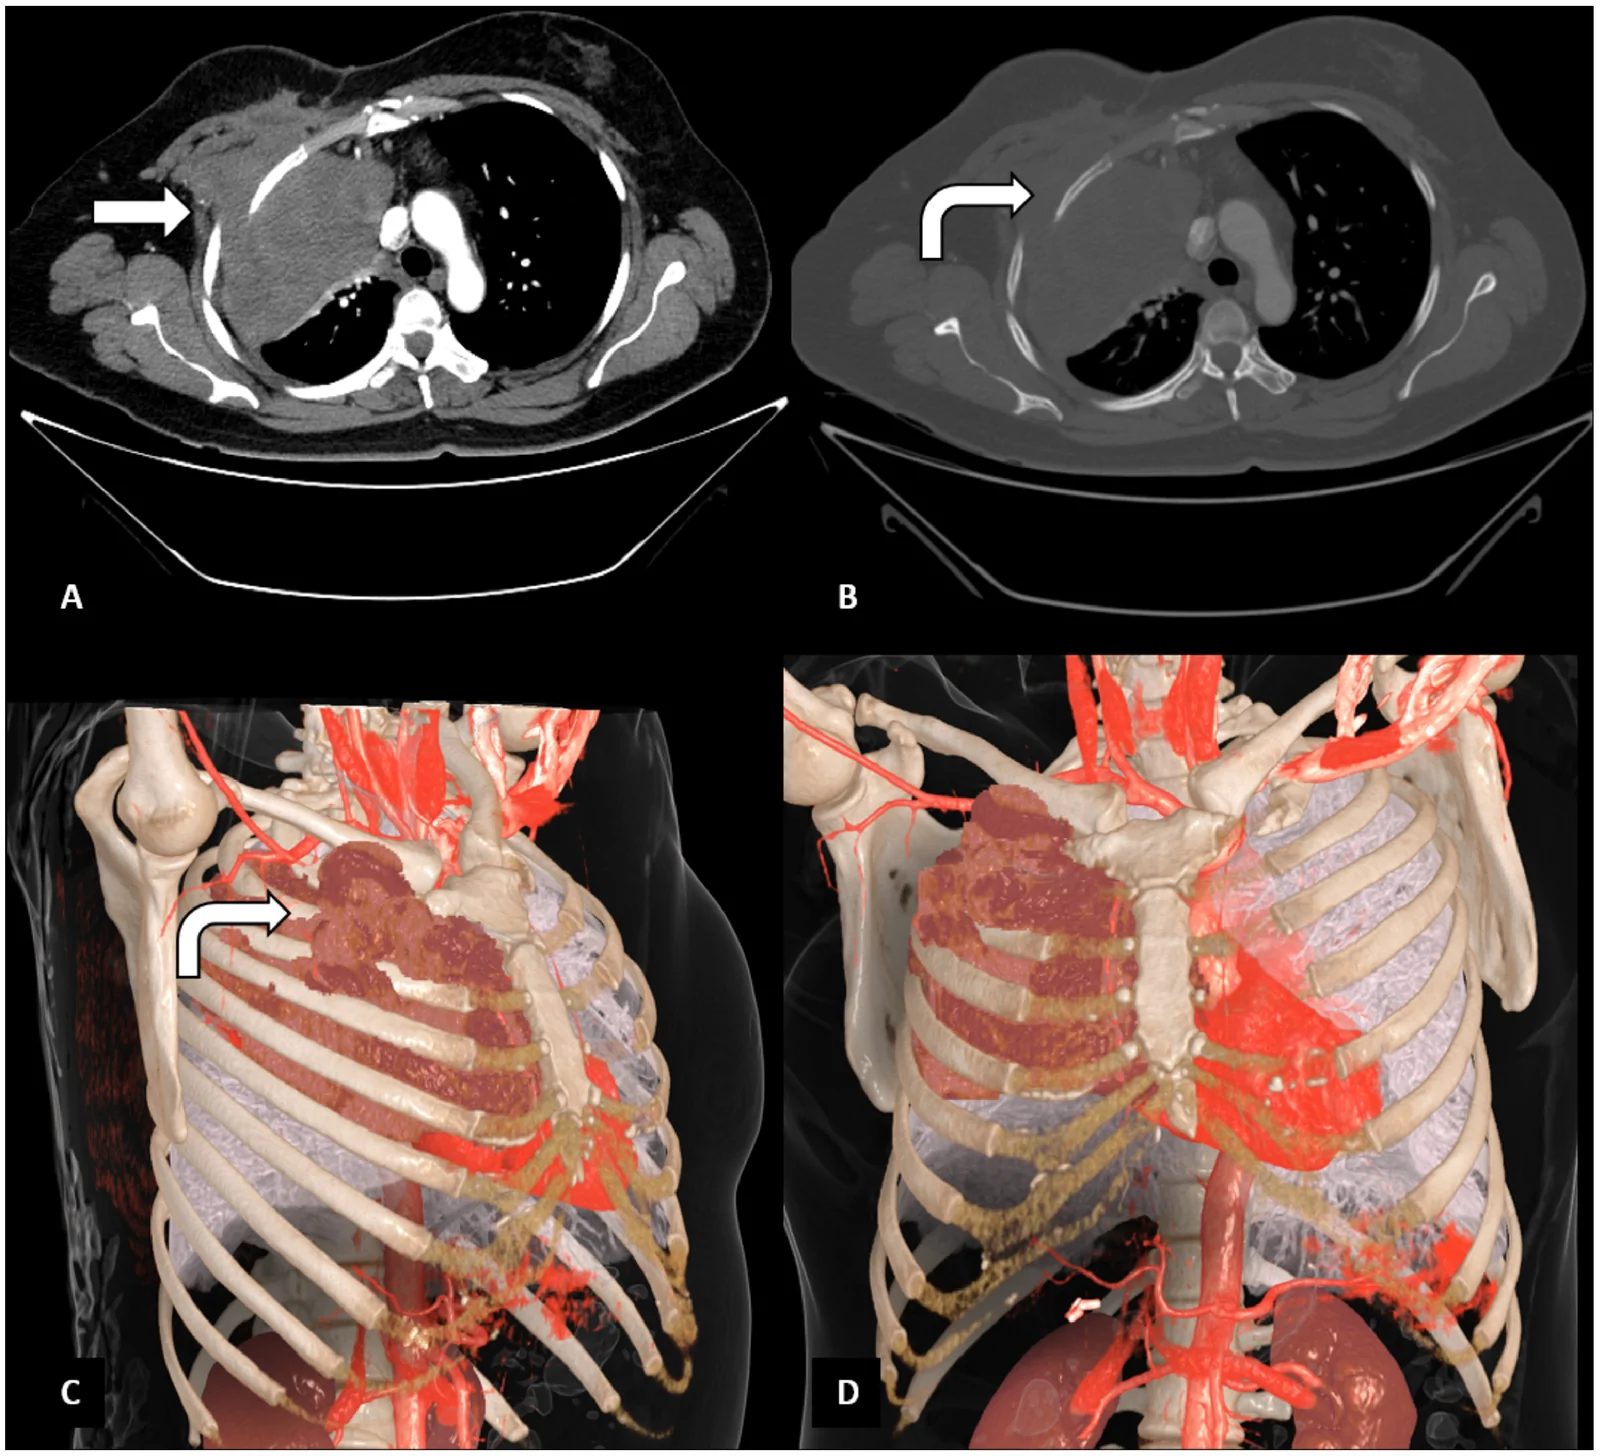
Arterial-phase contrast-enhanced chest CT showing chest wall involvement. (A) Axial soft-tissue window demonstrates tumor extension most prominent between the second and third costal arches (arrow). (B) Bone window shows preserved cortical bone without osseous destruction. (C and D) Oblique and coronal cinematic-rendering images demonstrate extrathoracic extension along the anterior chest wall (curved arrow).
